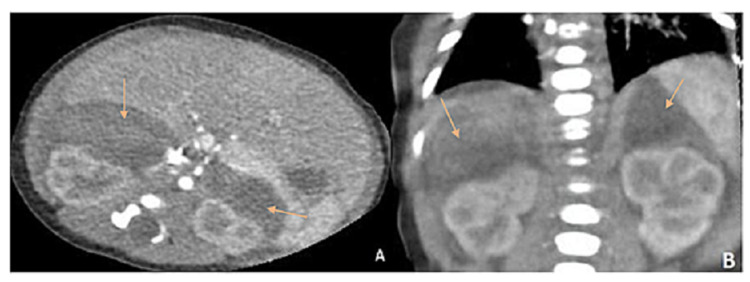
Findings of abdominal CT with contrast, showing bilateral adrenal hematomas Coronal (A) and sagittal (B) sections Arrows indicate bilateral adrenal hemorrhage
